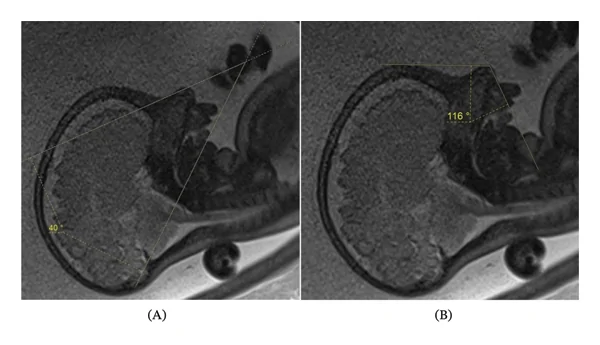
Prenatal biometric measurements of mandibular hypoplasia. (A) Fetal MRI demonstrating the inferior facial angle (IFA) of 40°, below the normal threshold of > 49°, consistent with severe micrognathia. (B) Sagittal T2‐weighted fetal MRI demonstrating the nasomental angle of 116°, significantly below the normal value of approximately 147°, further confirming severe mandibular deficiency.
